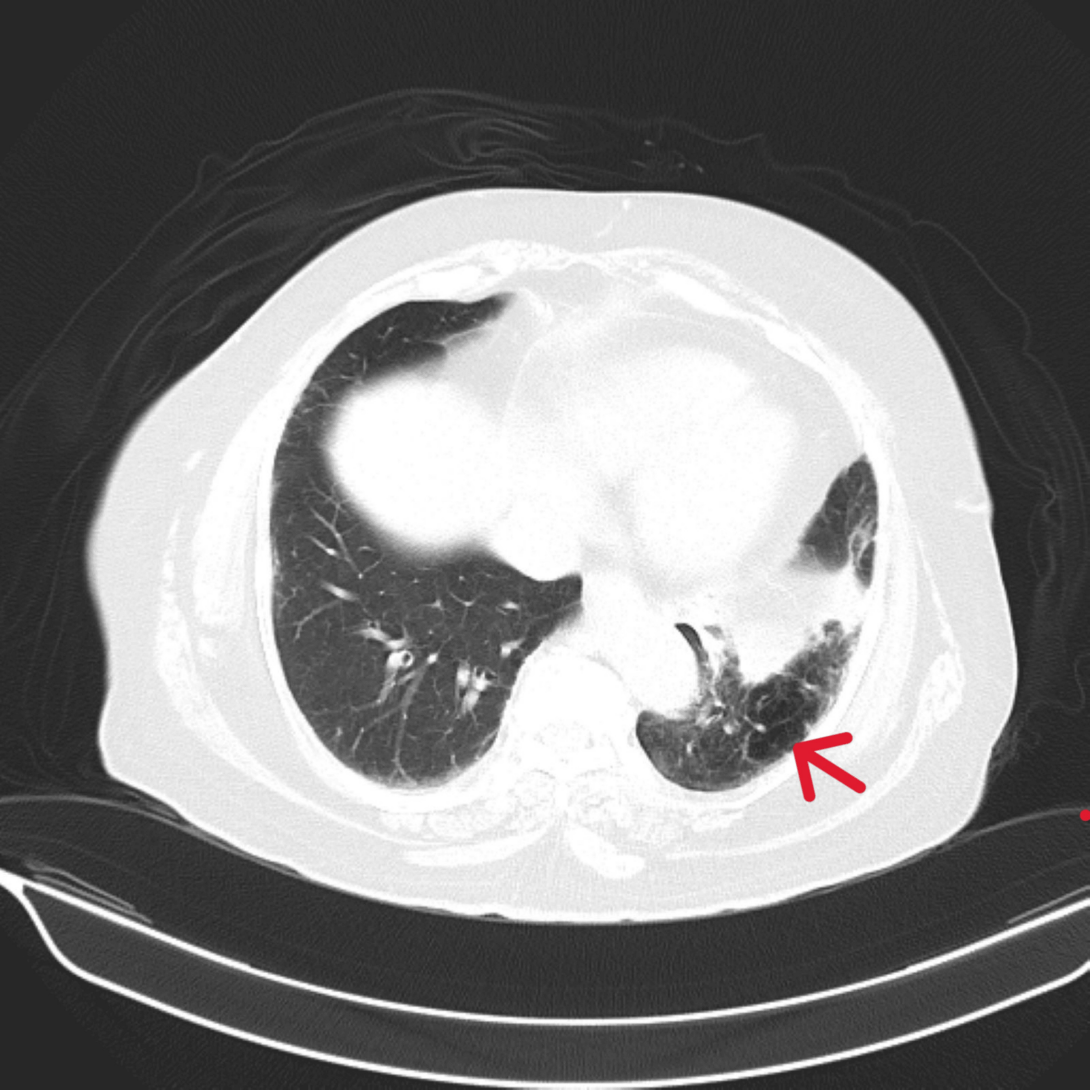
Axial section of a computed tomography (CT) scan of the chest Case 1: Volume loss with cystic bronchiectasis of left lower lobe (red arrow).
